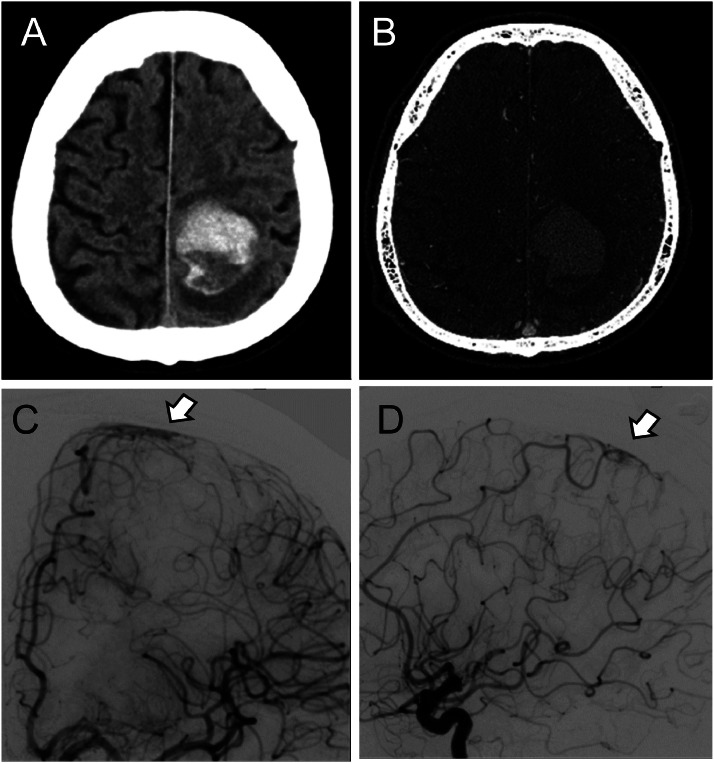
The images above (A, B) are axial CT scans of the head obtained upon admission. (A) CT demonstrating a subcortical hemorrhage (40 mm) in the left parietal lobe. (B) CTA showing no vascular abnormalities identified as the source of bleeding. The images below (C, D) are preoperative DSA. The left ICA angiograms in the anteroposterior (C) and lateral (D) projections reveal a fistula at the distal left anterior cerebral artery (arrowheads), suggesting a source of bleeding.
